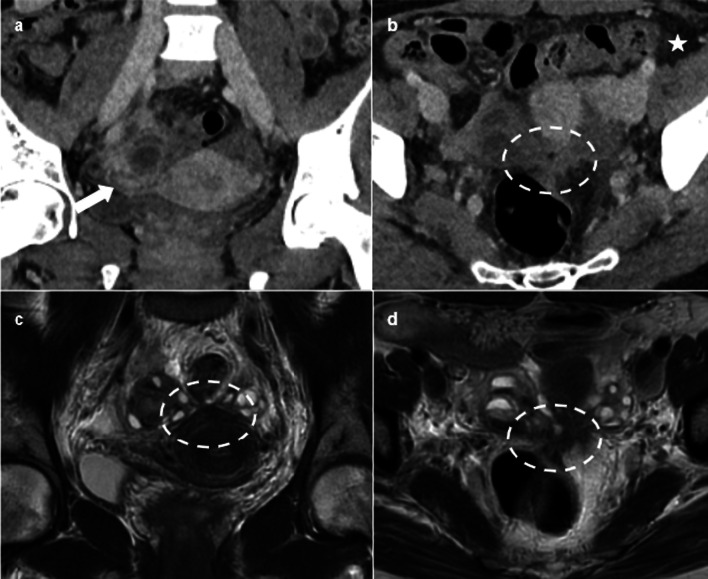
44-year-old woman presenting with pelvic pain and fever. CT (a, b) depicts signs of acute salpingitis, associating a strongly enhancing right fallopian tube (arrow) and pelvic fat stranding (star). On axial CT (b), the location of the ovaries is suggestive of deep endometriosis. T2W MRI (c, d) confirms the presence of an endometriotic nodule of the torus, responsible for a “kissing of the ovaries” (dotted circle)
